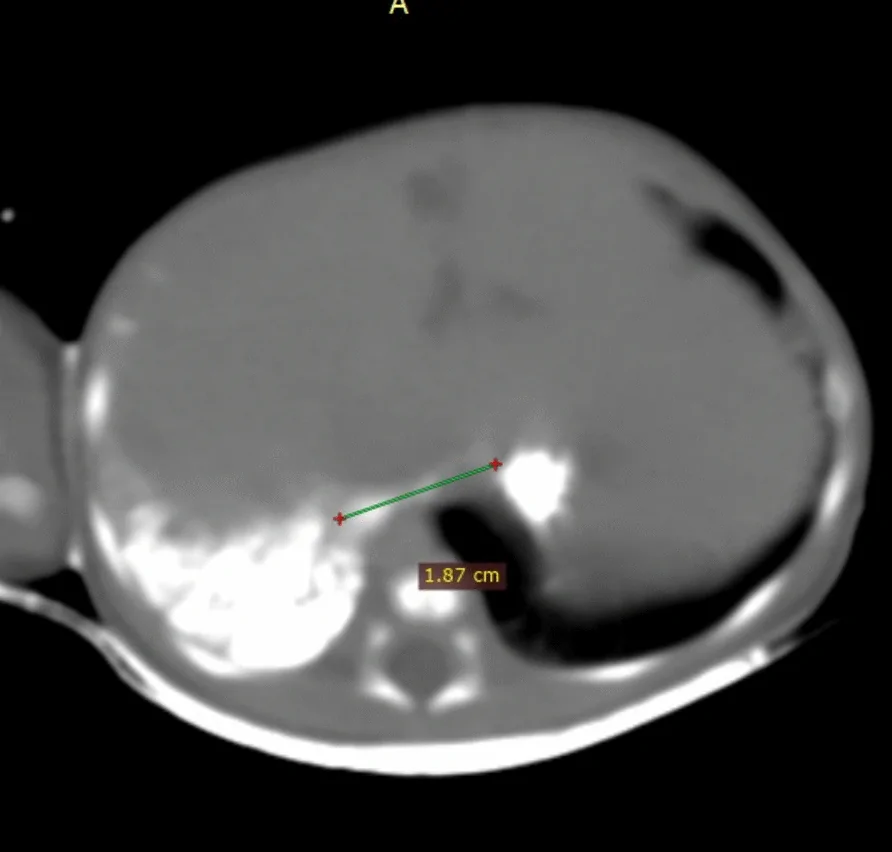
Axial computed tomography with Gastrografin contrast. Axial computed tomography following Gastrografin administration through the gastrostomy tube confirms a fistulous connection between the distal esophagus and an atretic right lung (arrow), with alveolar opacification due to contrast filling
